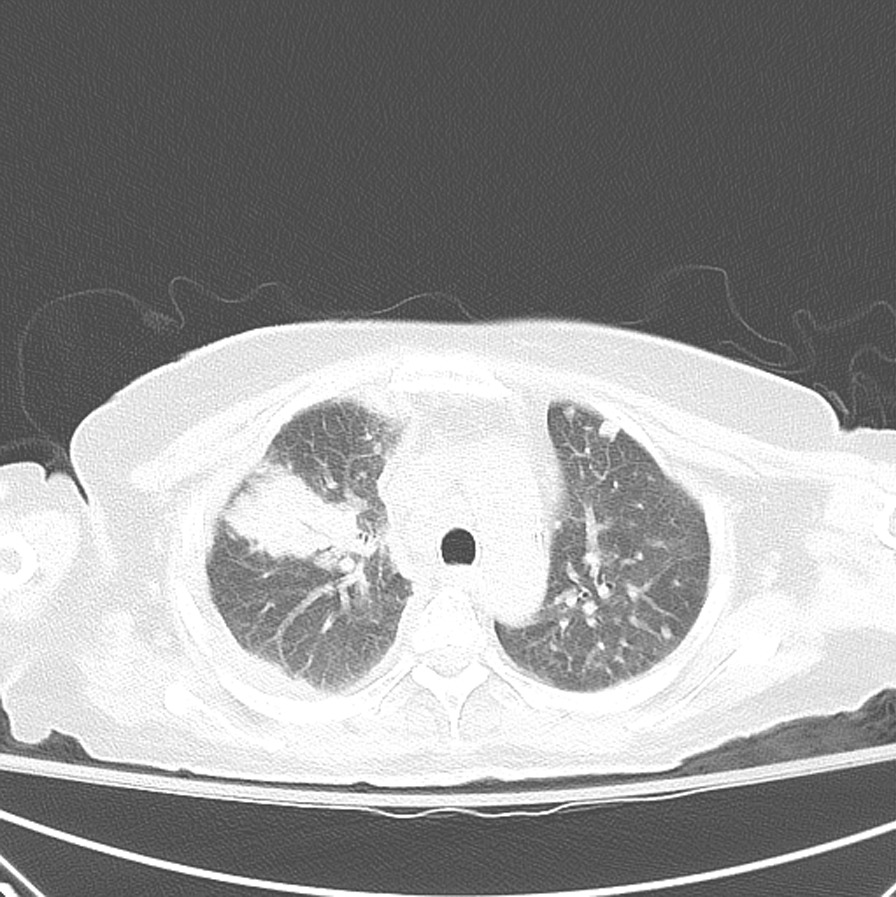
60 year-old female with acute coccidioidomycosis presenting with shortness of breath and chest pain. Right upper lobe consolidation, bilateral lung nodules, and partially imaged right pleural effusion are shown on this axial CT image of the lungs
